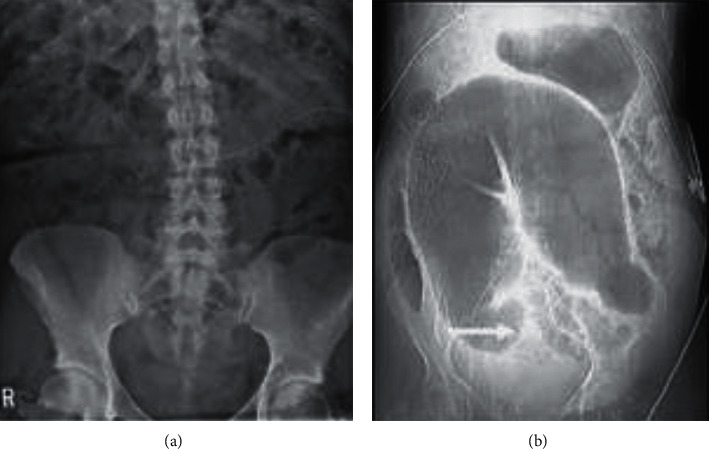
MRI functional constipation.
